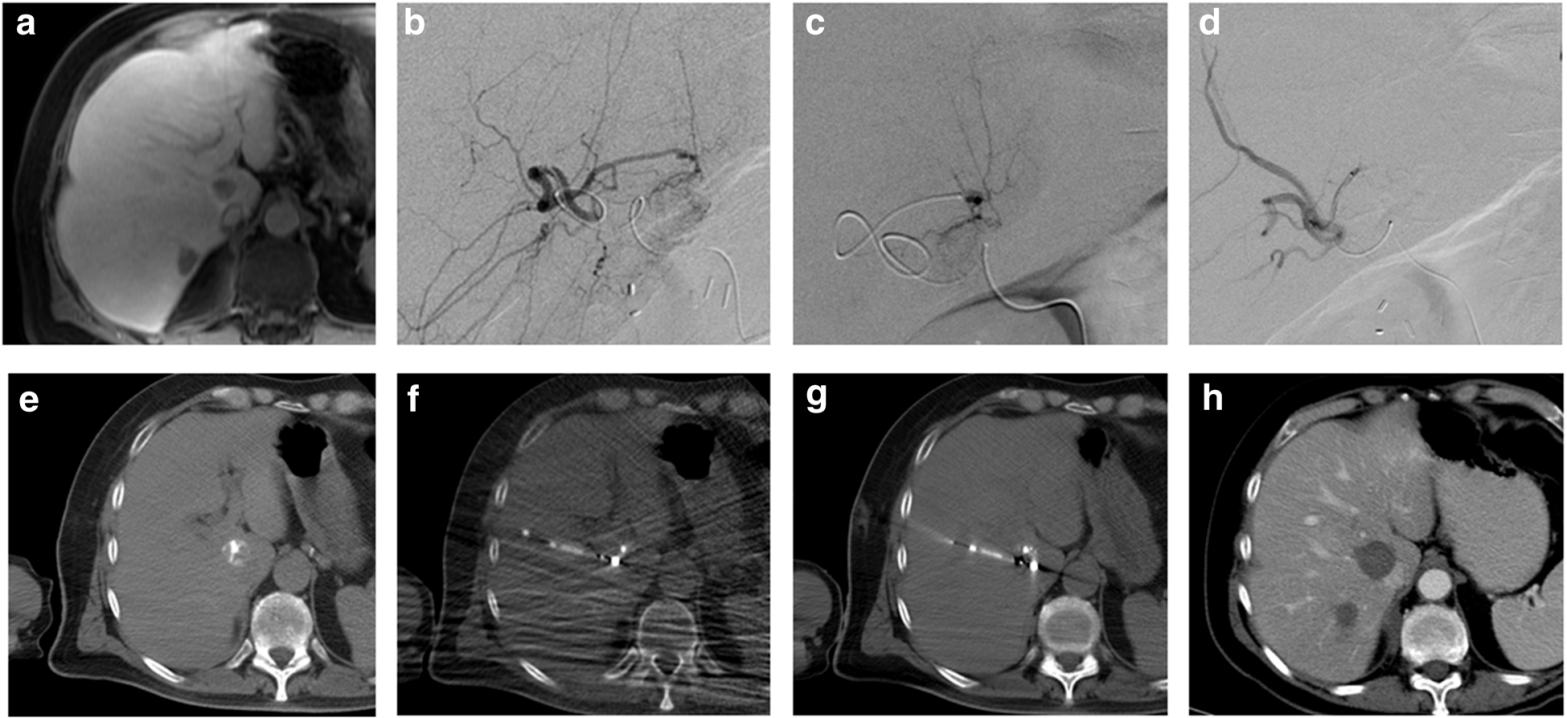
Example of liver ablation and embolization performed in an ACT room: The patient was a 61 year old male with 2 liver adrenal cortical carcinoma metastases in segment 7 (a) shown by the pre-operative contrast-enhanced MR (a). Selective bland embolization was performed under fluoroscopic guidance (b, c, d). The embolic agent facilitated tumor identification on the CT (e) and contributed to the guidance of the ablation probe under CT fluoroscopy (f). The probe treatment position was confirmed by a last CT before ablation (g). CT follow-up after 5 days show satisfying ablation margins (h)
